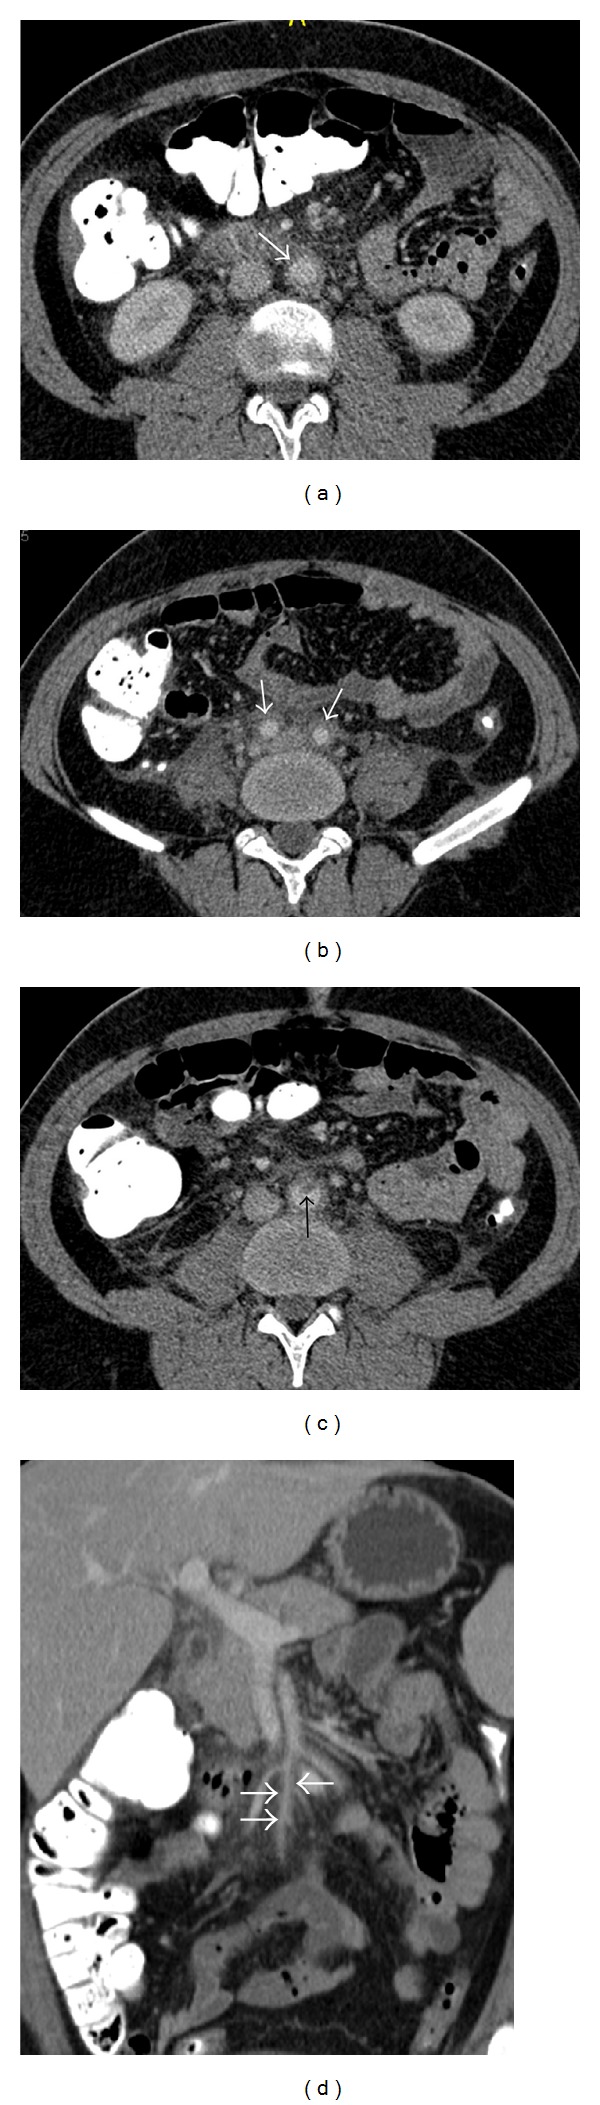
Axial CT angiographic images (a)–(c) show circumferential wall thickening in the abdominal aorta (arrow, (a)) and bilateral iliac arteries (arrows, (b)), as well as a small protruding atheroma in the abdominal aorta (arrow, (c)). Coronal image (d) demonstrates thickening of the wall of the superior mesenteric artery (arrows) and soft-tissue infiltration in the surrounding mesentery.
